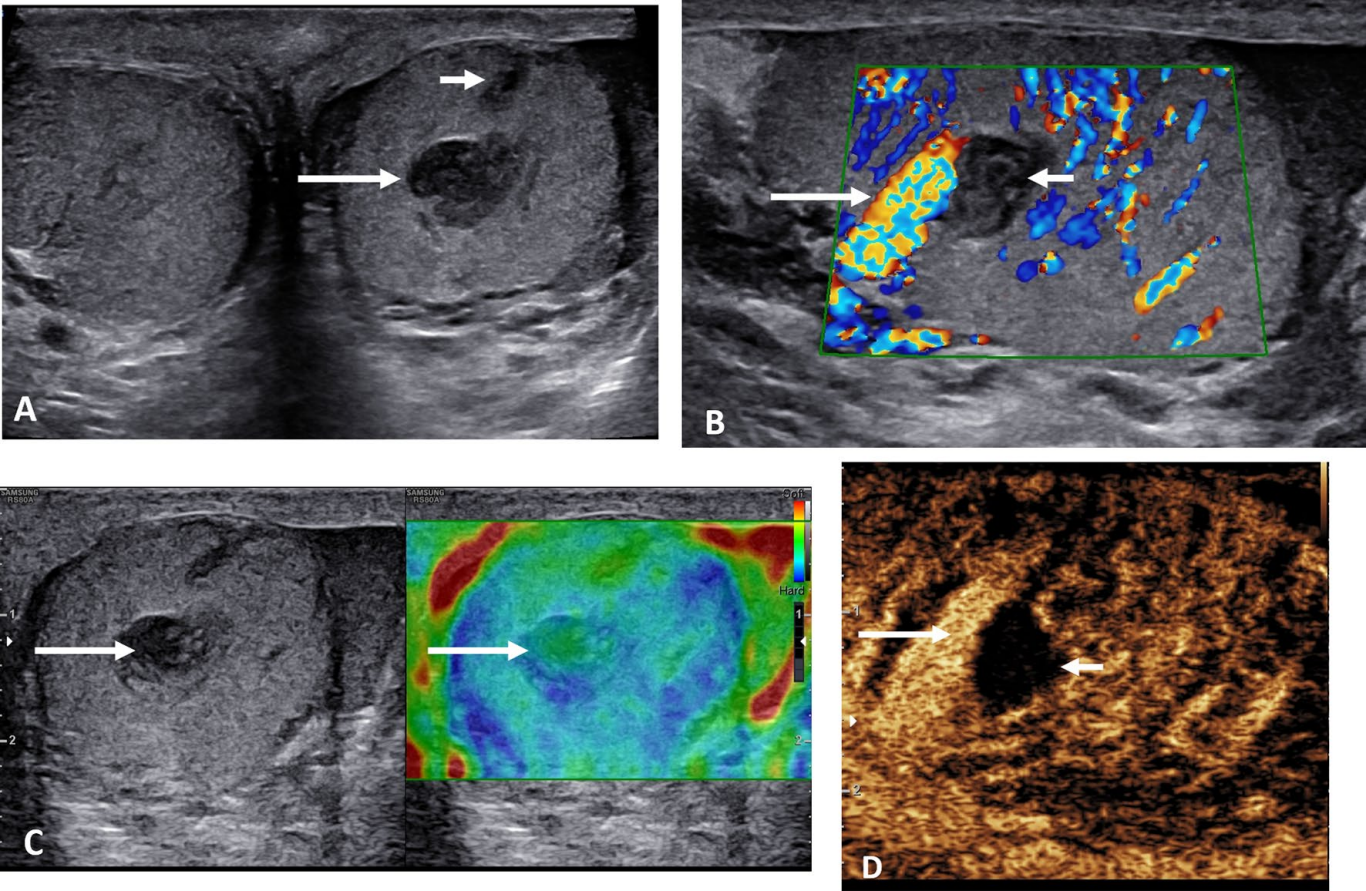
Post-biopsy pain, biopsy performed for investigation of infertility in a 45 year old, with an intra-testicular hematoma and arterio-venous fistula. A A transverse image on B-mode, demonstrating a focal low reflective lesion in the mid aspect of the left testis (long arrow). The biopsy tract is seen as a linear low reflective area (short arrow). B An oblique color Doppler ultrasound showing the presumedhaematoma (short arrow), with increased flow (long arrow) in a arterio-venous fistula. C On elastography the stiffness is intermediate (arrows), and will evolve with the stage of the liquefication of the hematoma. D On the contrast enhanced ultrasound examination, the hematoma (short arrow) is clearly depicted, with the arterio-venous fistula obvious (long arrow)
